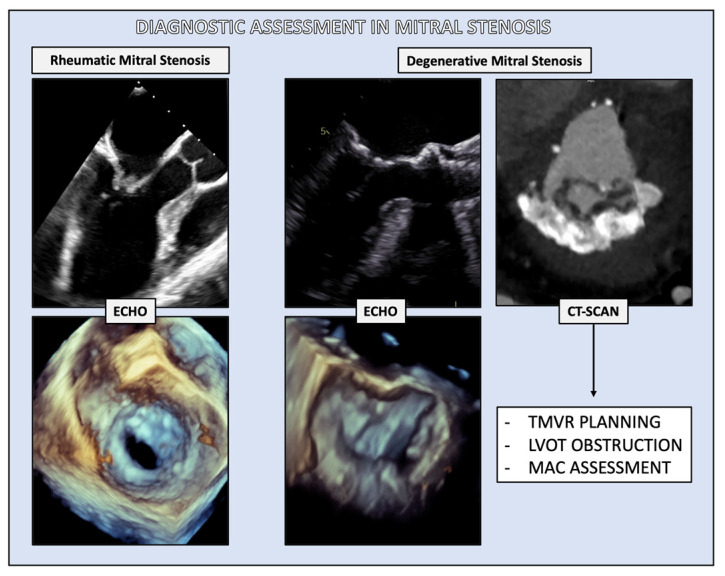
Diagnostic assessment in mitral stenosis. For rheumatic mitral stenosis, both transthoracic and transesophageal echocardiography serve as the primary diagnostic tools; in degenerative mitral stenosis, a CT scan is pivotal, primarily for the pre-procedural planning of a transcatheter procedure, as it could detect pitfalls, such as preventing left ventricle outflow tract obstruction.
